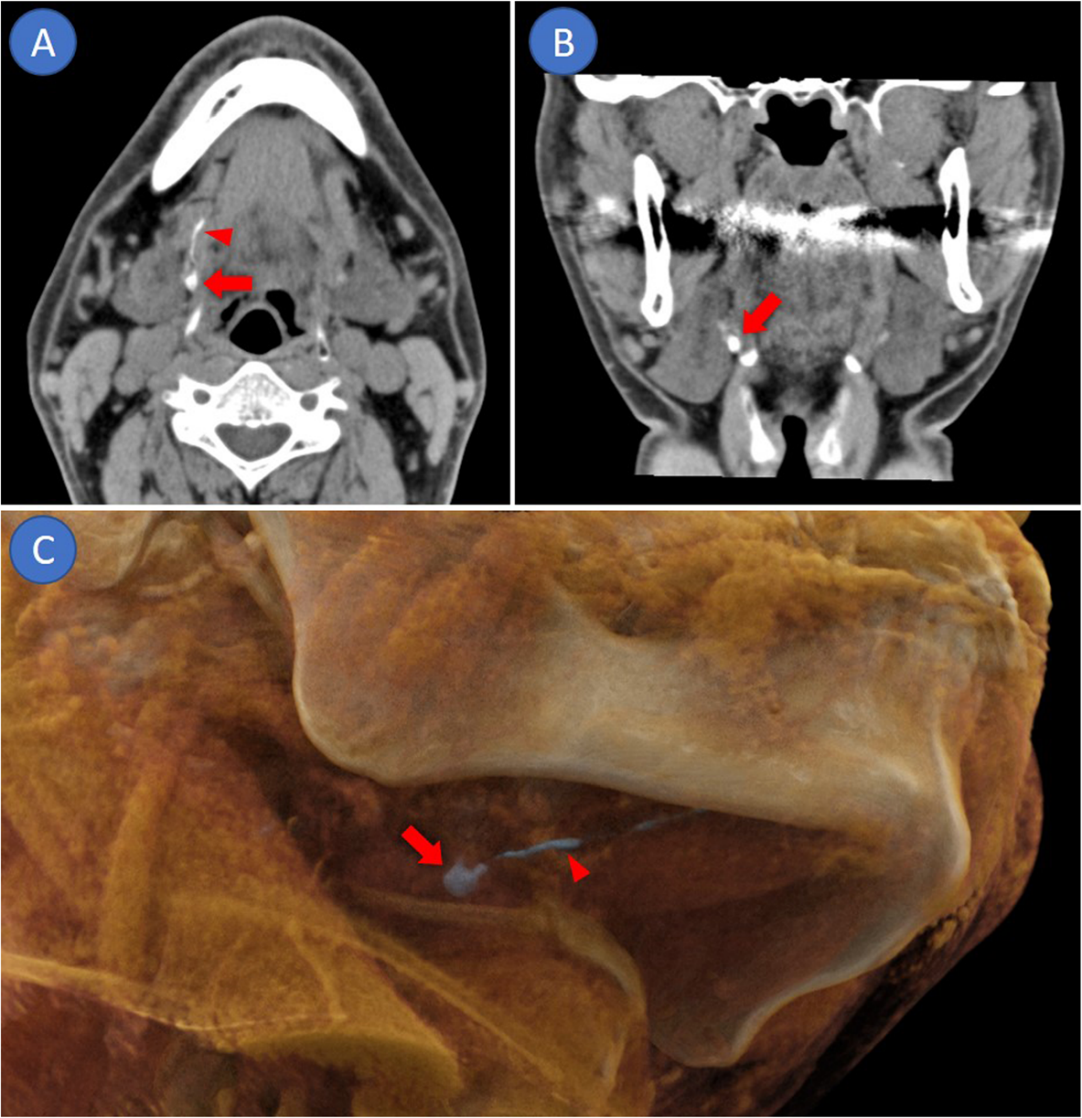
CT lymphography. a: Axial image, b: coronal image, c: 3D image with cinematic volume rendering technique. Arrows indicate sentinel lymph node. Arrowheads indicate lymphatics
